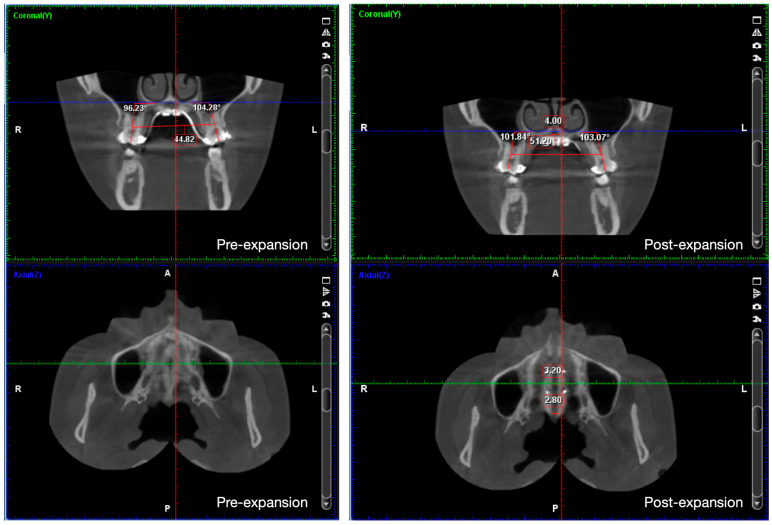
Pre- and post-expansion CBCT images and measures. Molar inclinations were not worsened after MARPE. Expansion pattern was fairly parallel from anterior to posterior on the axial view. (Red line: Sagittal plane; green line: Coronal plane; blue line: Axial plane).
